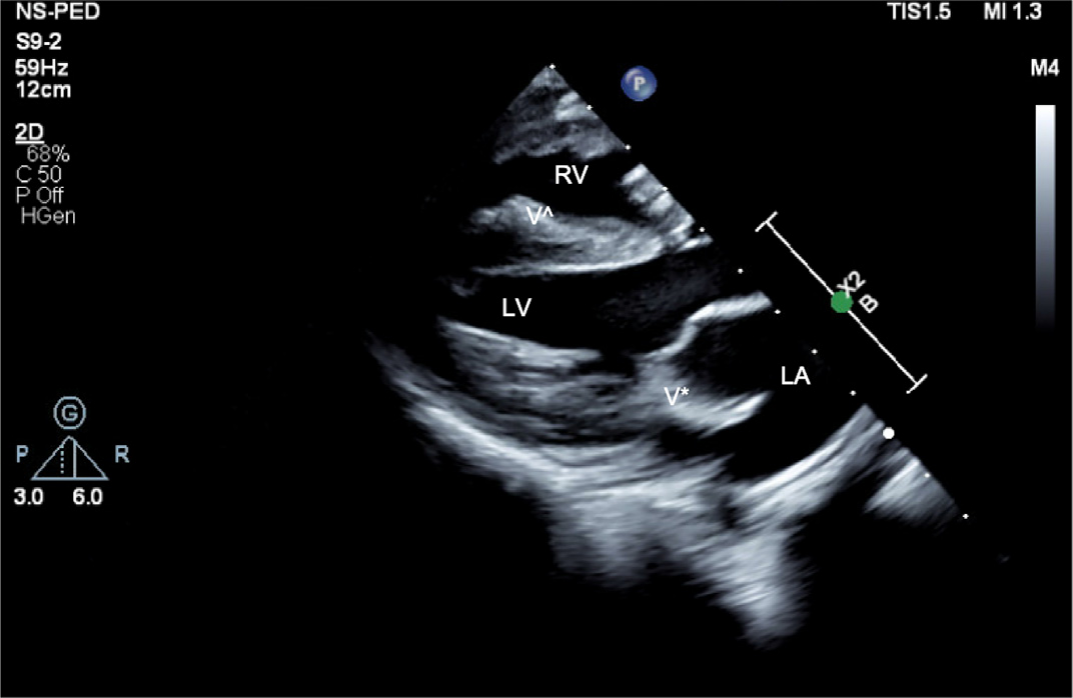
Echocardiogram of Vegetations: Mitral Valve and Right Ventricle Transthoracic parasternal long-axis view demonstrating the 24 × 10-mm vegetation (V∗) on the mitral valve. Another vegetation (Vˆ) is seen in the right ventricular outflow tract adhering to the septum, in proximity to ventricular septal defect. Abbreviations as in Figure 1.
